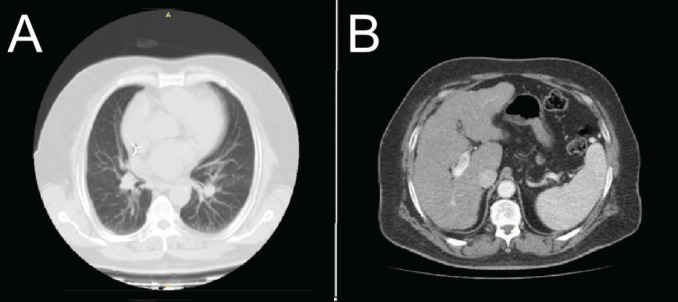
CT chest and abdomen scan with contrast, axial slices (a): Lung window shows no focal pleuropulmonary lesion; (b): Abdomen shows irregular liver borders with no focal mass lesion.
